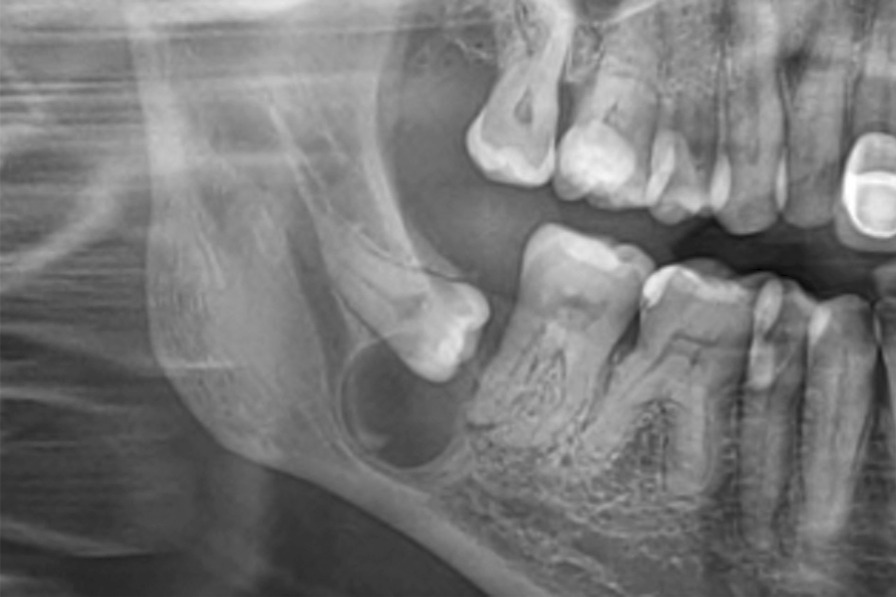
Case 2: Initial orthopantomogram revealing a round, well-circumscribed radiolucency with a diameter of about 1 cm around the lower part of the crown of the impacted horizontal tooth #48
